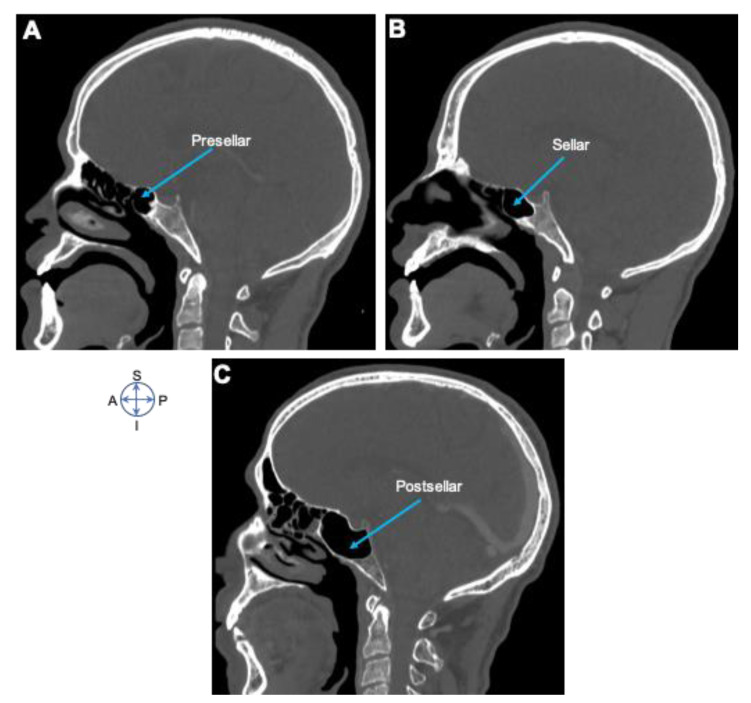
Anatomographic variants of sphenoid sinus. Sagittal CT images showing (A) presellar, (B) sellar, and (C) postsellar types of sphenoid sinus. Note that the sellar type of pneumatization was found to be the most frequent anatomographic variant of sphenoid sinus in the Ethiopian population. Image orientation: S, superior; I, inferior; A, anterior; P, posterior.
